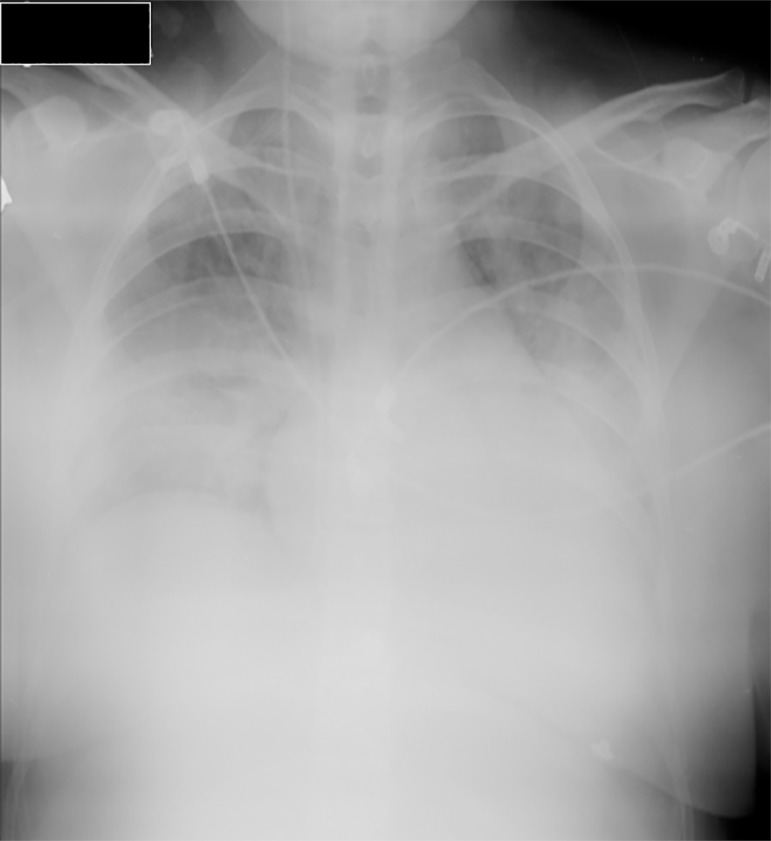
Chest X-ray (frontal view) on admission. Note the bilateral volume loss
